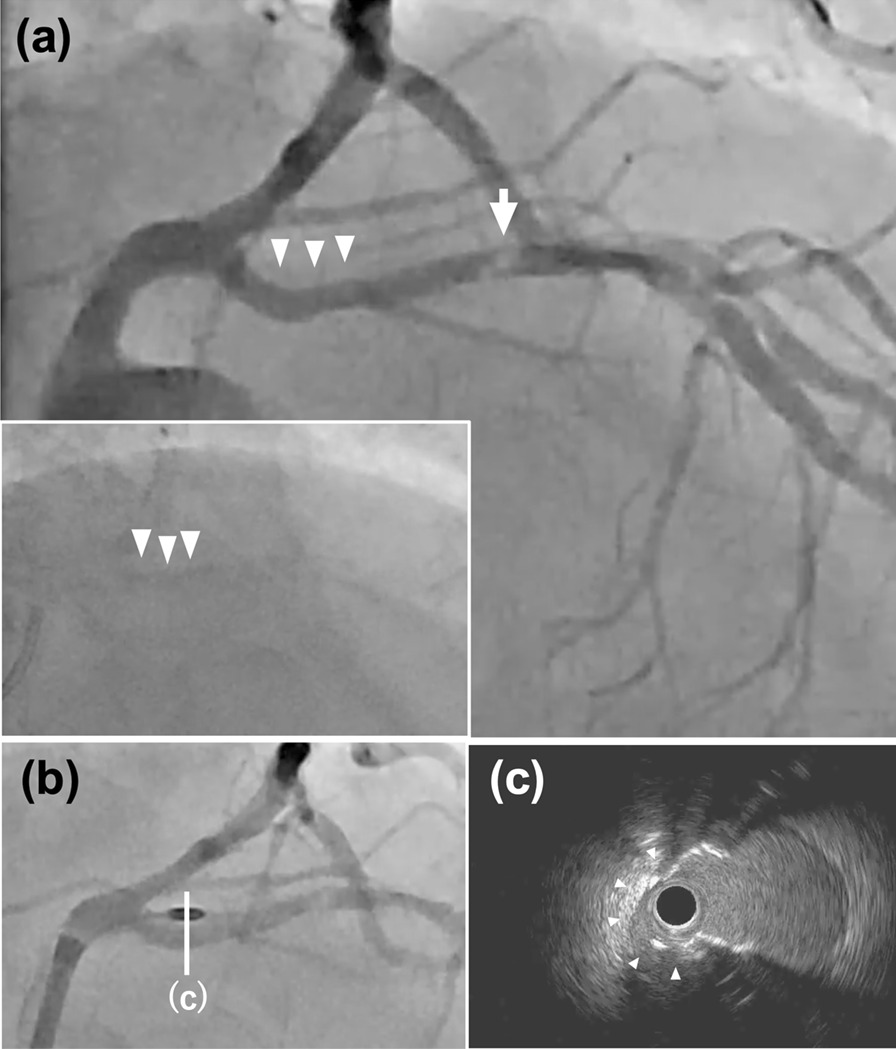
Coronary angiography and intravascular ultrasound (IVUS) imaging. a Coronary angiography before rotational atherectomy showing a tortuous calcified lesion (arrowheads) and a calcified nodule (arrow). b Rotational atherectomy. Drilling with a 1.5-mm eccentric burr (arrow). c IVUS view (b, white line) showing eccentric medial cutting at 9 o’clock (arrowheads)
